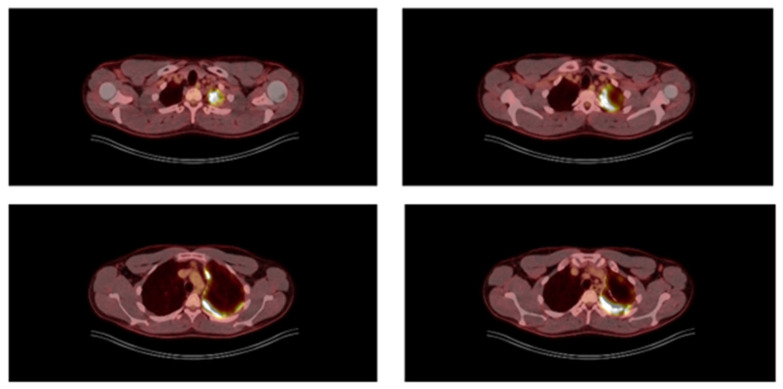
The preoperative staging ^18^FDG PET/CT scan aimed at detection of mediastinal lymphadenopathy and extra-thoracic metastatic sites. There were no such findings but the previously pleurodesis (left hemithorax) was pathologically avid even one year post talcage. This was erroneously attributed to the pleurodesis procedure but was in fact misdiagnosed pleural aspergillosis and was even unrecognized during the metastatectomy procedure.
